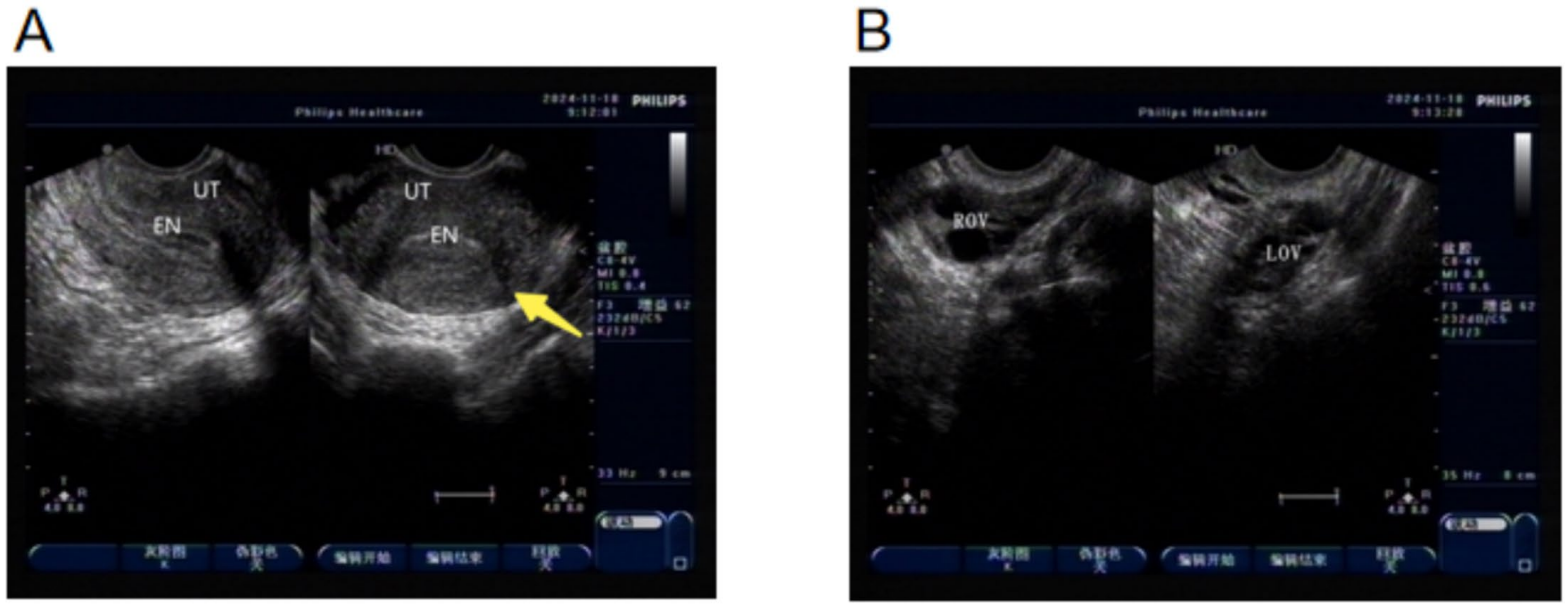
Ultrasound images on the day of admission. (A) Yellow arrow: no pregnancy lesion seen in the left interstitial tubal region. (B) Bilateral ovaries.
